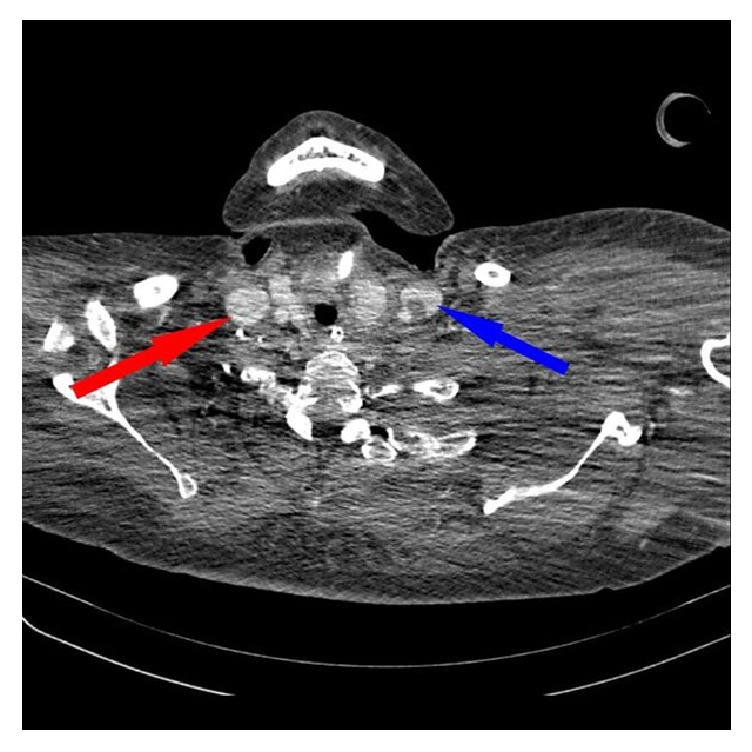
CT scan (axial image) of the thorax with a contrast enhancing agent. Results with thrombus adhering to the wall in both internal jugular veins (left IJV, blue arrow, and right IJV, red arrow).
